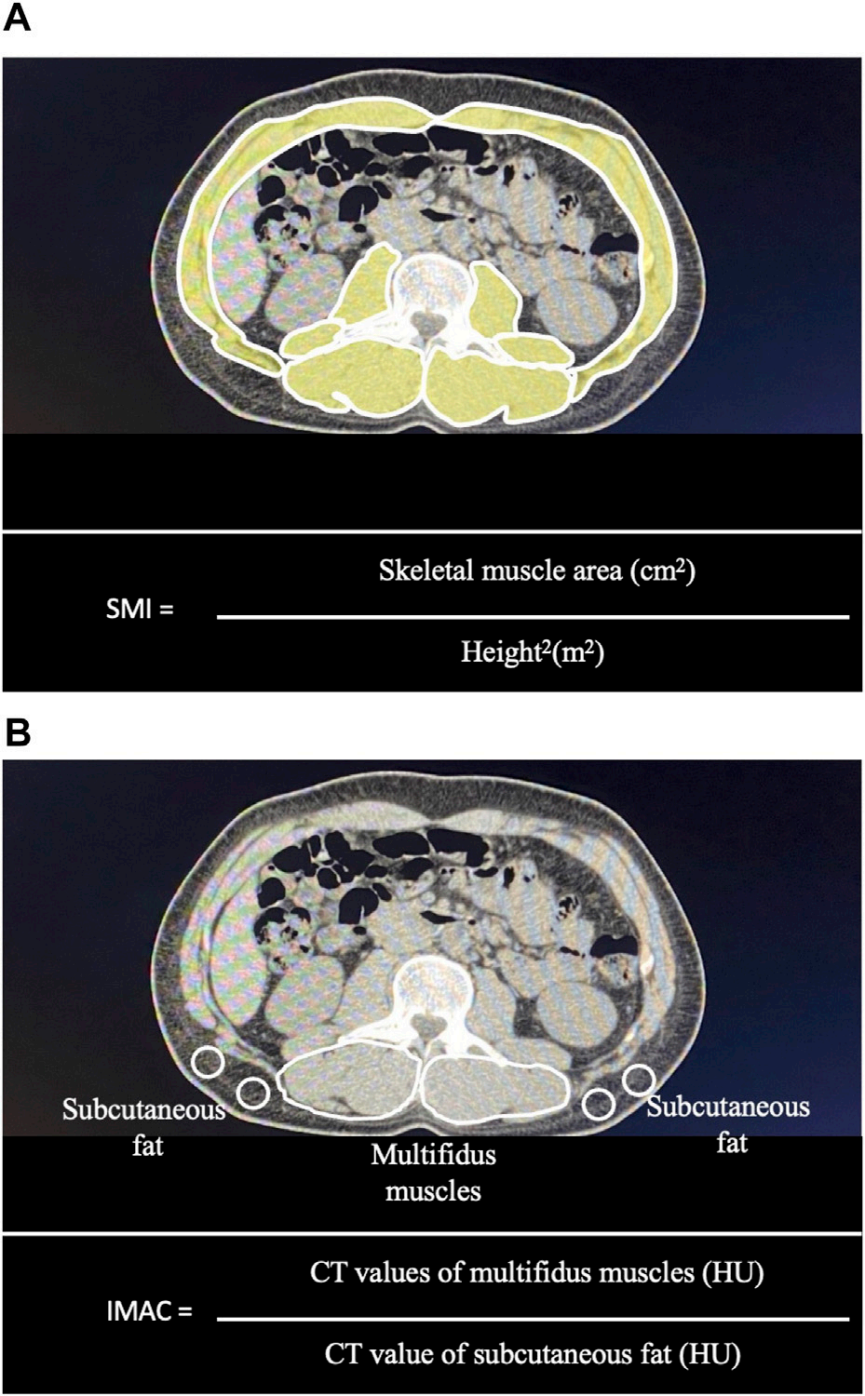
Measurement of the skeletal muscle mass index (SMI) and intramuscular adipose tissue content (IMAC) using a cross-sectional CT image obtained at the third lumbar vertebral level: (A) Skeletal muscle areas were measured by manual tracing. Skeletal muscle areas were normalized with patient height in m^2^ and expressed as the SMI. (B) The preoperative mean CT value for the right and left multifidus muscles (in Hounsfield units, HU) was divided by the mean CT value for four points of subcutaneous fat and expressed as the IMAC.
